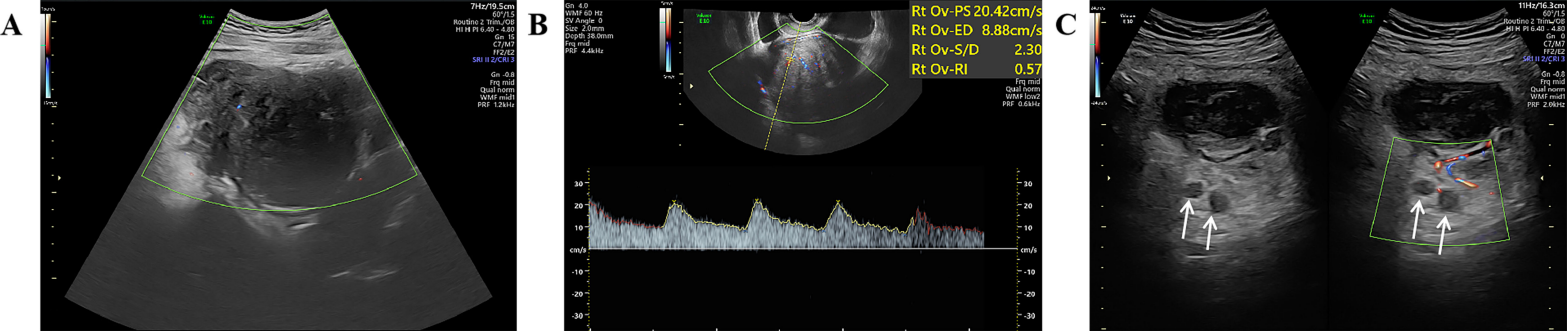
Ultrasound images; (A) Color Doppler showing punctate blood flow signals inside the hypoechoic mass; (B) Pulsed Doppler spectrum inside the hypoechoic mass; (C) Enlarged right abdominal lymph node (white arrow).
